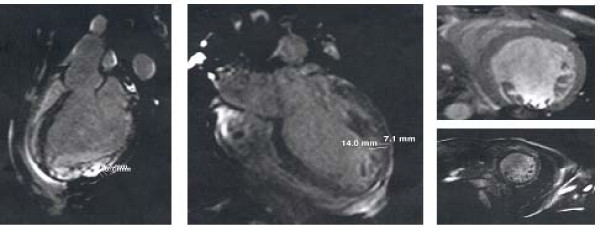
Cardiac Magnetic Resonance Imaging confirming IVNC. Numerous proeminent trabeculae and deep intertrabecular recesses. Ratio between distance from the epicardial surface to the trough of the trabecular recess/distance from the epicardial surface to peak of trabeculation = 6,7/19,2 (left image). Two-layered myocardium, ratio non-compacted endocardium to compacted epicardium = 2 (middle image).
